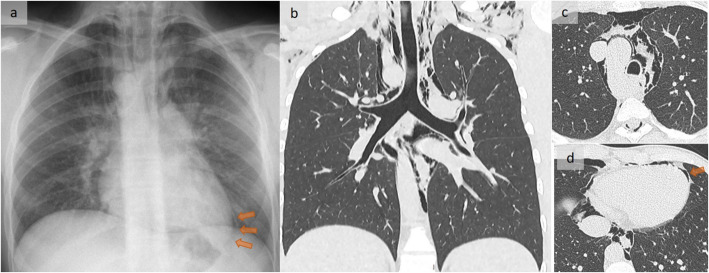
Atraumatic pneumomediastinum. A patient with sudden spontaneous retrosternal and interscapular pain. Frontal chest X-ray (a), coronal oblique reformation in lung windowing (b), and axial slices (c, d) show gas outlining the mediastinal structures, with pneumopericardium (orange arrows in a and d) appearing as a left paracardiac subtle line in a and subcutaneous emphysema. Note the right aortic arch. Conservative management led to a favorable outcome
